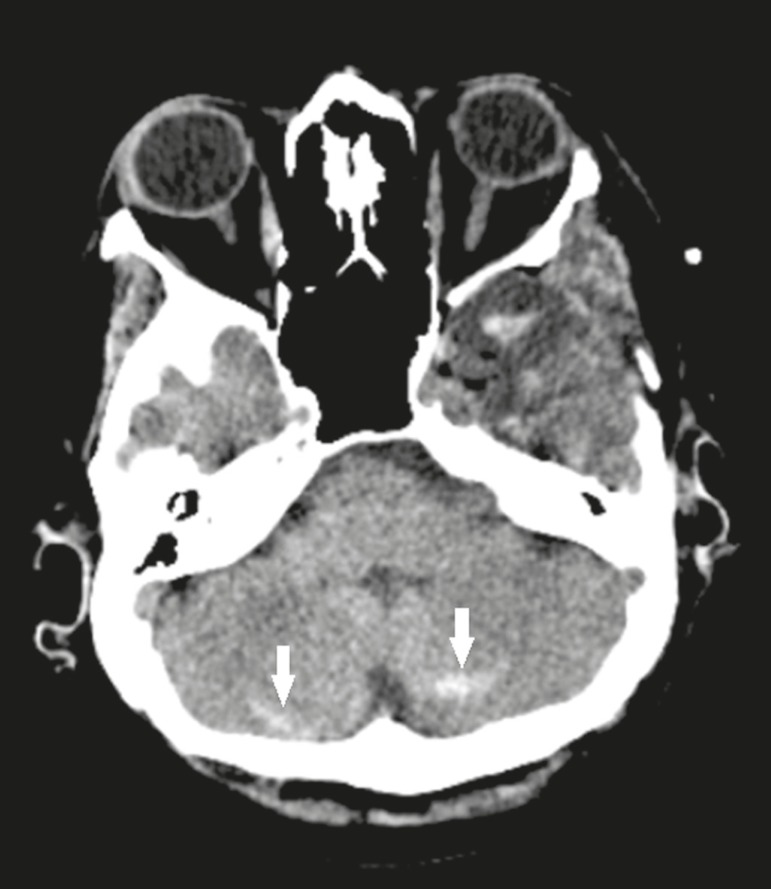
Remote cerebellar hemorrhage. Axial CT, with a parenchymal
